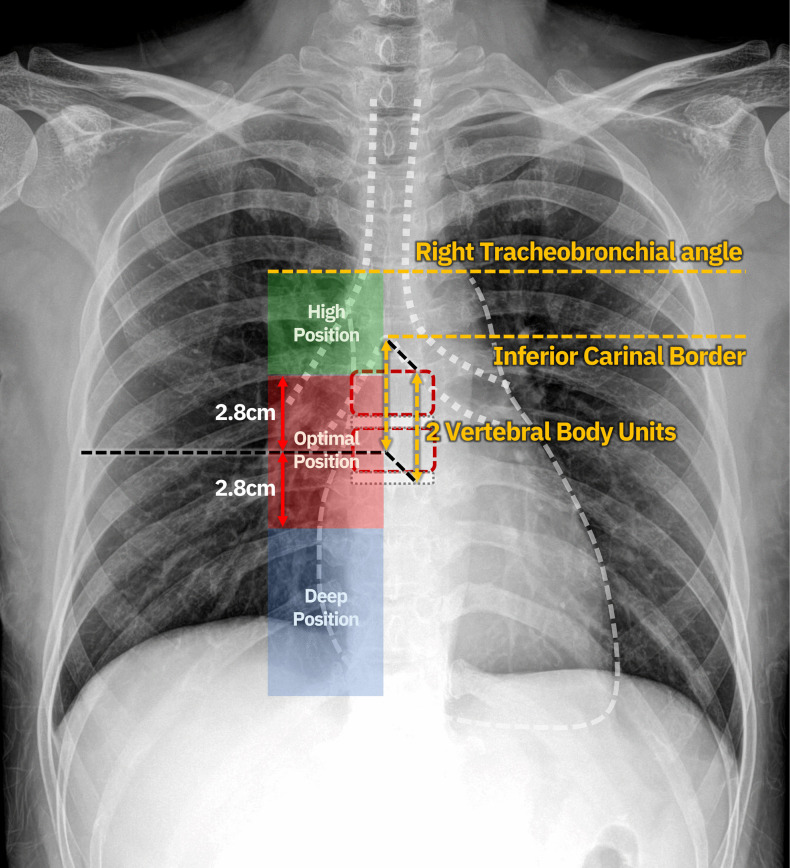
Definition of optimal, high, and deep PICC tip positions on anteroposterior chest radiography. The cavoatrial junction (CAJ) is defined as 2 VBU below the inferior carina border. Optimal position (red zone) is the catheter tip located within ±2.8 cm of the designated CAJ. High position (green zone) indicates the tip located in the superior vena cava between the optimal zone and the right tracheobronchial angle. Deep position (blue zone) indicates the tip located in the right atrium below the optimal zone. CAJ: cavoatrial junction; VBU: vertebral body unit.
